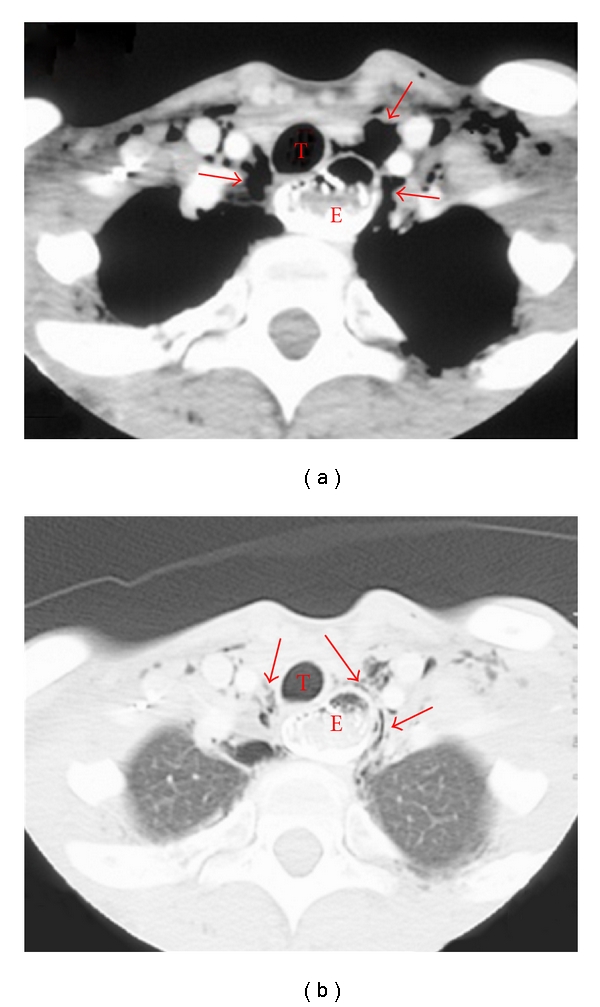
(a) A CT showing a dilated esophagus (labeled “E”) and pneumomediastinum (shown with the arrows). There is air, fluid, and solid food within the esophagus. The trachea is labeled “T” for reference. (b) Another CT scan showing the dilated esophagus and the pneumomediastinum.
